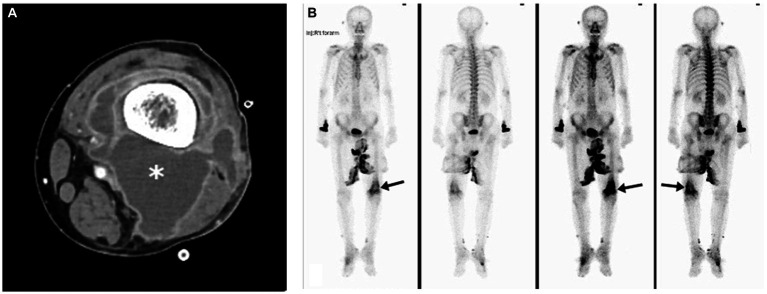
Melioidosis presented with septic arthritis of case 9. Contrast-enhanced axial computed tomography image showed marginal enhancing abscess-like accumulation in the right knee joint (asterisk) (A). Tc-99 m MDP bone scan revealed marked-degree increased radiotracer uptake in the corresponding region (arrows) (B).
